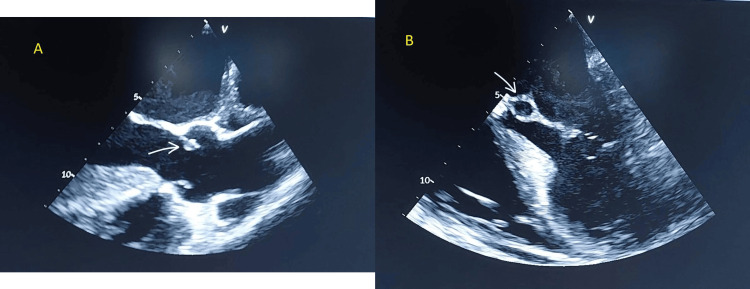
Transesophageal echocardiography (TEE) scan, showing (A) aortic valve vegetation (arrow), and (B) aortic root abscess (arrow), another complication of infective endocarditis and a risk factor for poor prognosis.
